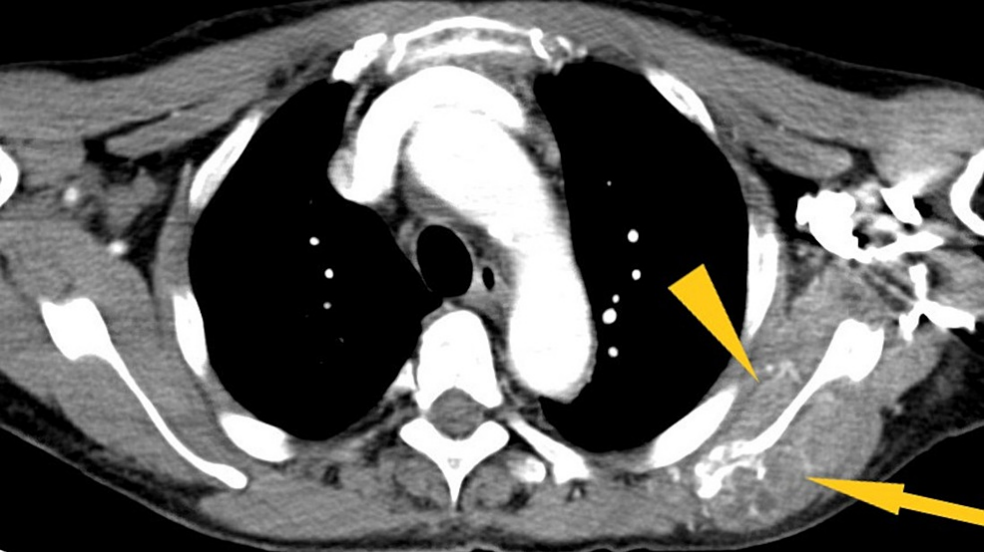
CT chest with contrast shows expansile, extrahepatic hepatocellular carcinoma metastasis to the left scapula (yellow triangle and arrow)
